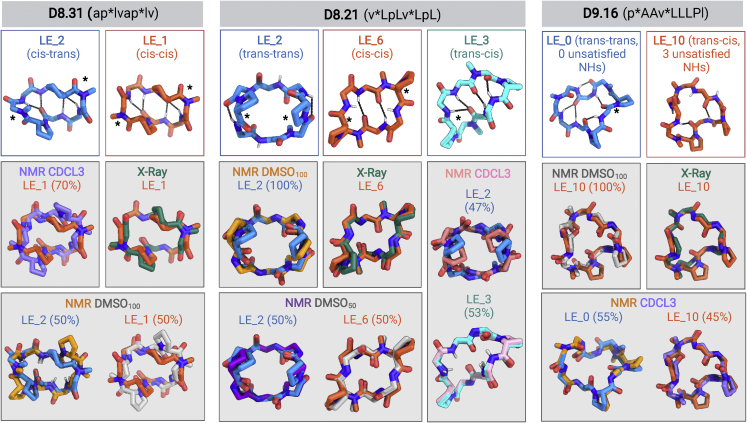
Design and structural characterization of conformation switching macrocycles Design models and experimentally determined structures (X-ray and NMR) for different conformational states of designs D8.31 (left), D8.21 (middle), and D9.16 (right). The design model and predicted low-energy states are shown in the top row. The superposition between the predicted low-energy states and the experimental structures is shown in the gray boxes. The solvent conditions for the NMR structures and low-energy states in superposition plots are indicated by similar colors in the labels and structures. In conditions with multiple conformations, the relative percentage of each conformation in the solution is also indicated. All three designs show solvent-dependent changes in the populations and switching between at least two different conformations. The conformational switch in D8.31 does not change the number of unsatisfied NHs, but for both D8.21 and D9.16, the states with fewer unsatisfied NHs are favored in the nonpolar solvents. See also Tables S3 and S4, and Figure S6, Figure S7, Figure S8, Figure S9, Figure S10.
